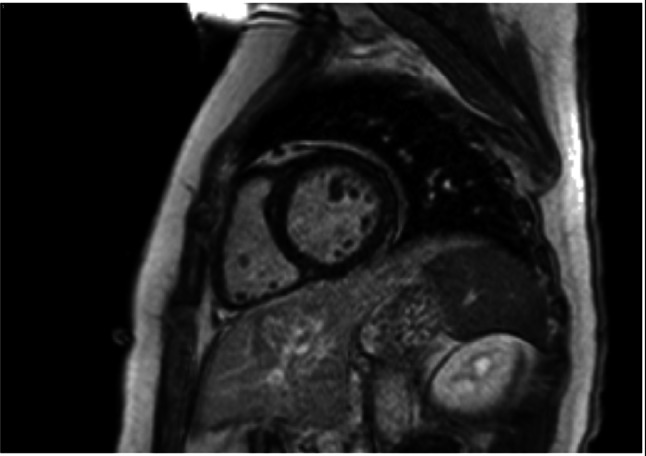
Cardiac MRI short axis view demonstrating epicardial late gadolinium enhancement located mainly in the lateral wall, consistent with non-ischemic injury, most likely myocarditis. There is also associated small pericardial effusion
